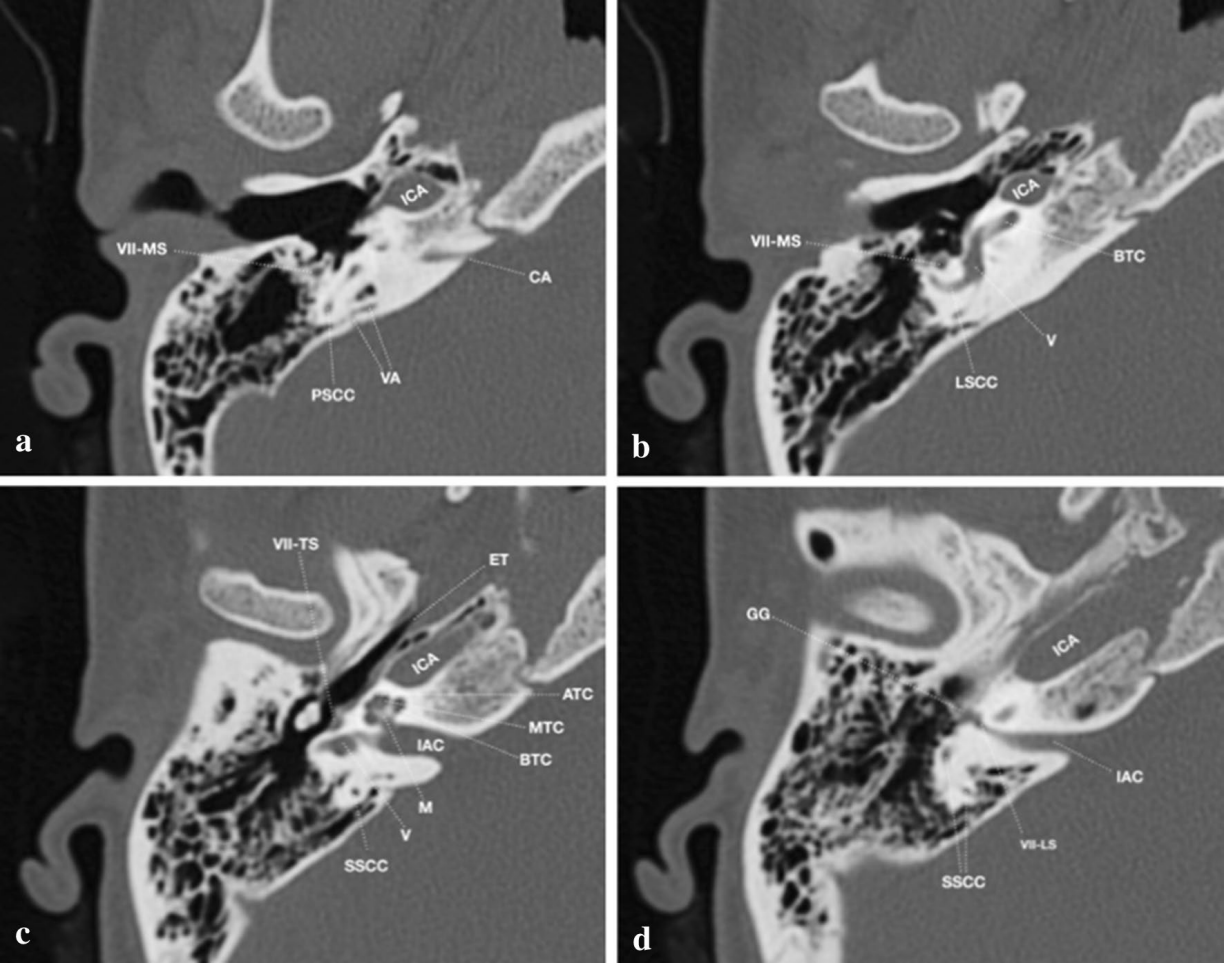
Normal anatomy of Inner Ear structures in high-resolution CT (selection of 0.625-mm thick axial slices); caudocranial direction from a to d. Legend: ATC: apical turn cochlea; BTC: basal turn cochlea; MTC: middle turn cochlea; CA: cochlear aqueduct; ET: eustachian tube (entrance); IAC: internal auditory canal; ICA: internal carotid artery; M: malleus; LSCC: lateral semicircular canal; PSCC: posterior semicircular canal; SSCC: superior semicircular canal; V: vestibule; VA: vestibular aqueduct; VII: 7th cranial nerve (LS: labyrinthine segment; MS: mastoid segment; TS: tympanic segment); GG: geniculate ganglion
